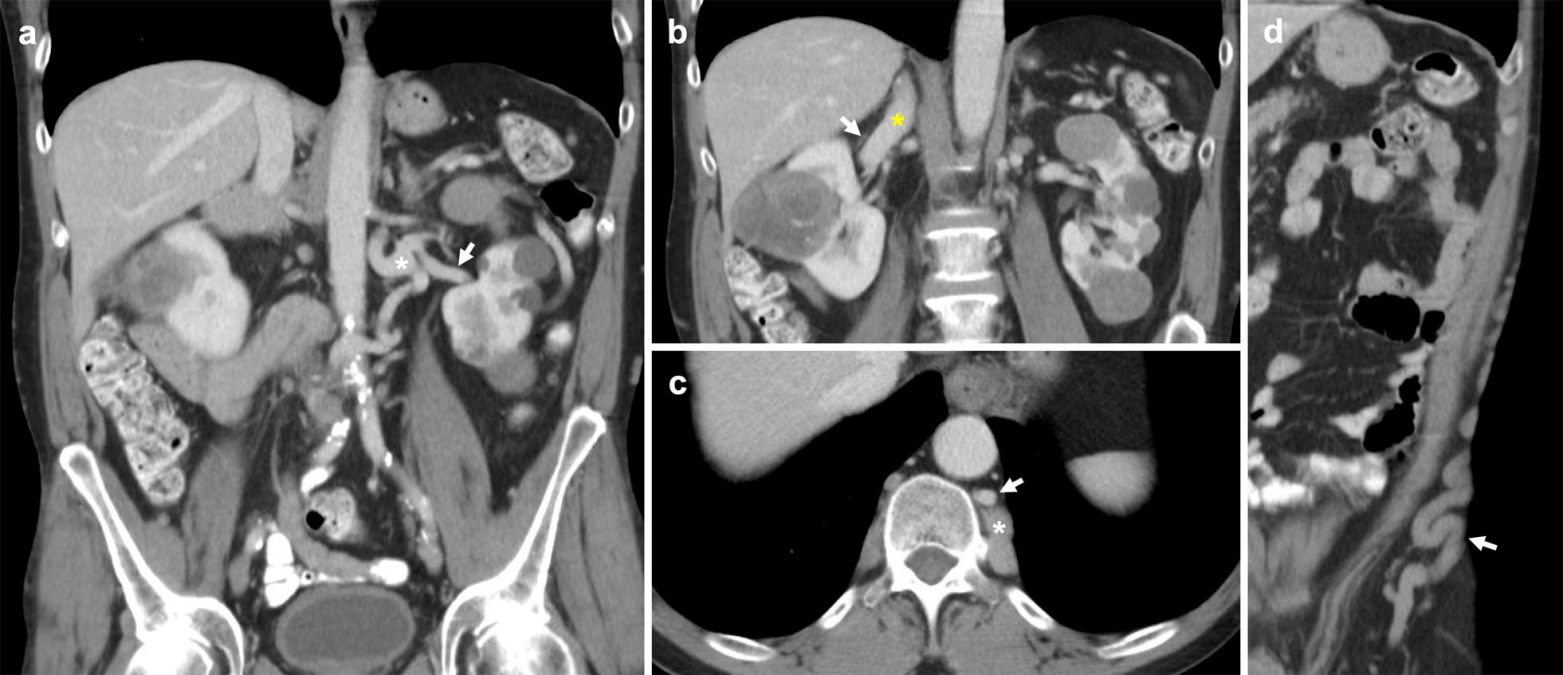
Interrupted IVC (renal level) with azygos and hemiazygos continuation. Anomaly detected incidentally on imaging for a 55-year-old man with biopsy proven renal cell carcinoma. The paired common iliac veins continue as bilateral ascending lumbar veins draining into the azygos–hemiazygos system. a Contrast-enhanced coronal CT shows the left renal vein (arrow) draining into a tortuous paravertebral collateral (asterisk). b The right renal vein (arrow) joins the suprarenal IVC (asterisk). c Axial CT image depicts a left-sided paravertebral collateral (arrow) that drains into the hemiazygos vein (asterisk) sited posteriorly. d Coronal CT image with a prominent lateral abdominal wall collateral vein
